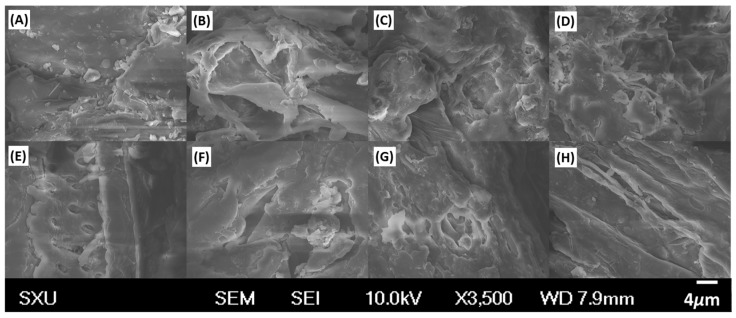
SEM graphics of Radix *Salviae miltiorrhizae* samples. (A) Raw materials, (B) extracted by DES-based microwave-assisted, (C) 75% methanol-based microwave-assisted extraction, (D) water-based microwave-assisted extraction, (E) DES-based ultrasound-assisted treatment. (F) 75% methanol-based ultrasound-assisted extraction, (G) DES-based hot reflux-assisted extraction, and (H) 75% methanol-based hot reflux-assisted extraction.
